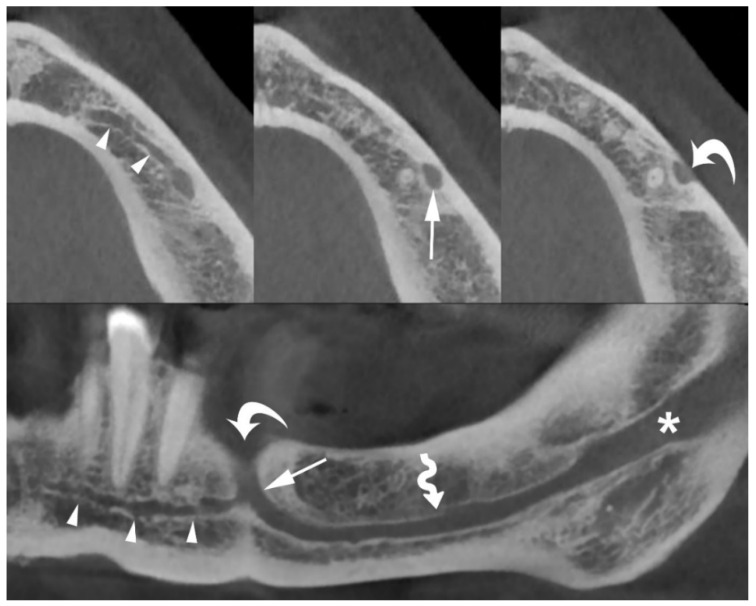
Axial (top) and panoramic (bottom) cone-beam computed tomography (CBCT) images of the left hemi-mandible demonstrate that the mandibular incisive canal (MIC) (arrowheads) originates from the anterior loop of mandibular canal (arrows) and proceeds towards the midline below the apices of the teeth. The mental foramen (curved arrows), mandibular canal (wavy arrow), and mandibular foramen (asterisk) are also shown.
